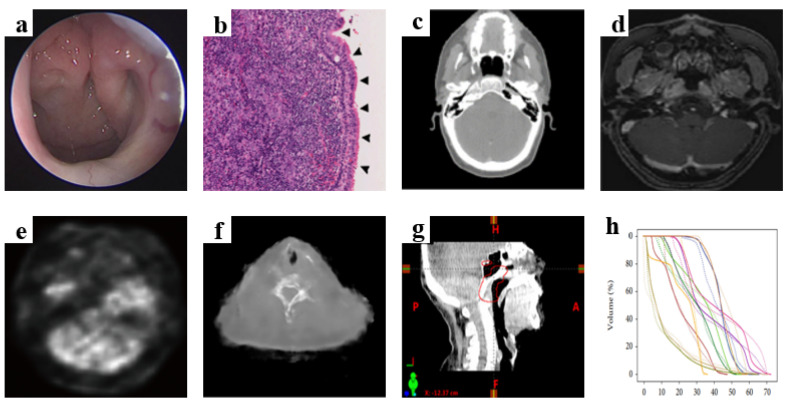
Sample images from various datasets: (a) endoscopic image (Mohammed et al., 2020 [26]); (b) whole slide image (Chuang et al., 2020 [31]); (c) CT image (Daoud et al., 2019 [48]); (d) MRI image (Guo et al., 2020 [58]); (e) PET image (Zhao et al., 2019 [43]); (f) CT-MR image (Wang et al., 2019 [38]); (g) CBCT-CT image (Li et al., 2019 [49]); (h) DVH image (Zhuang et al., 2021 [67]).
